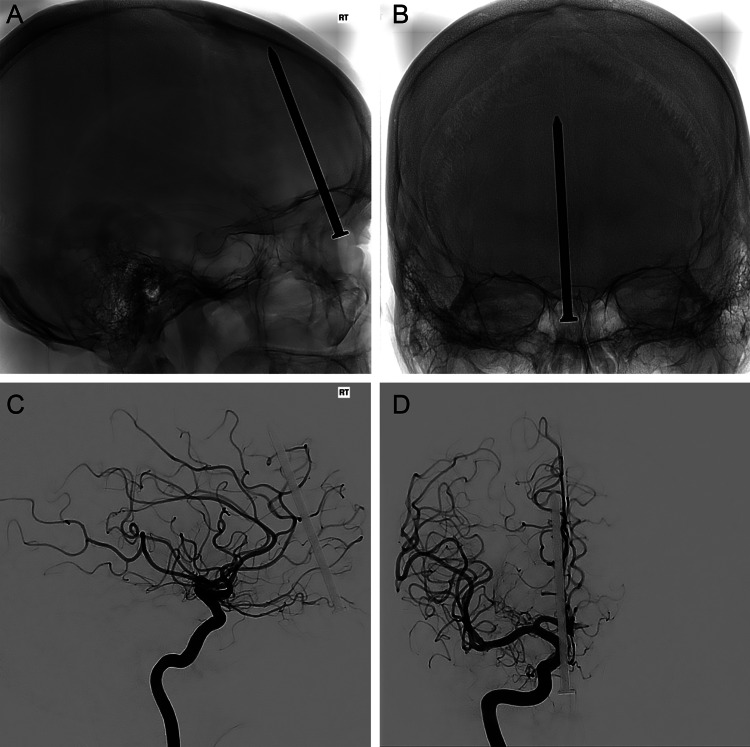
Preoperative radiographic and angiographic imaging showing the absence of vascular injury or intracranial hemorrhage. Preoperative imaging demonstrated no aneurysm, pseudoaneurysm, vascular injury, arteriovenous shunting, or active extravasation. Unsubtracted (A) lateral and (B) anteroposterior radiographs of the skull demonstrating the sagittal and coronal projection of the nail, respectively. Digitally subtracted (C) lateral and (D) posteroanterior angiography demonstrating a silhouette of the nail and no obvious vascular injury after right internal carotid artery contrast injection. *Used with permission from Barrow Neurological Institute, Phoenix, Arizona.*
